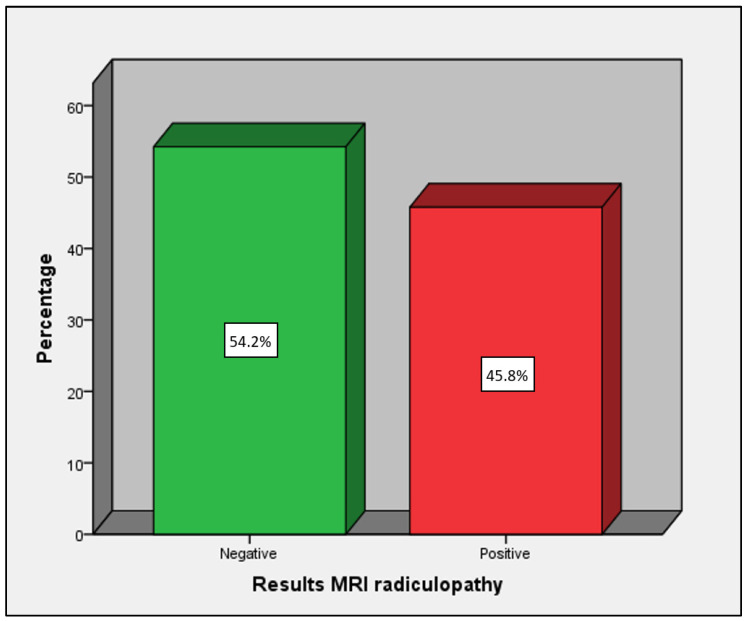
Results of MRI diagnosis of radiculopathy.
